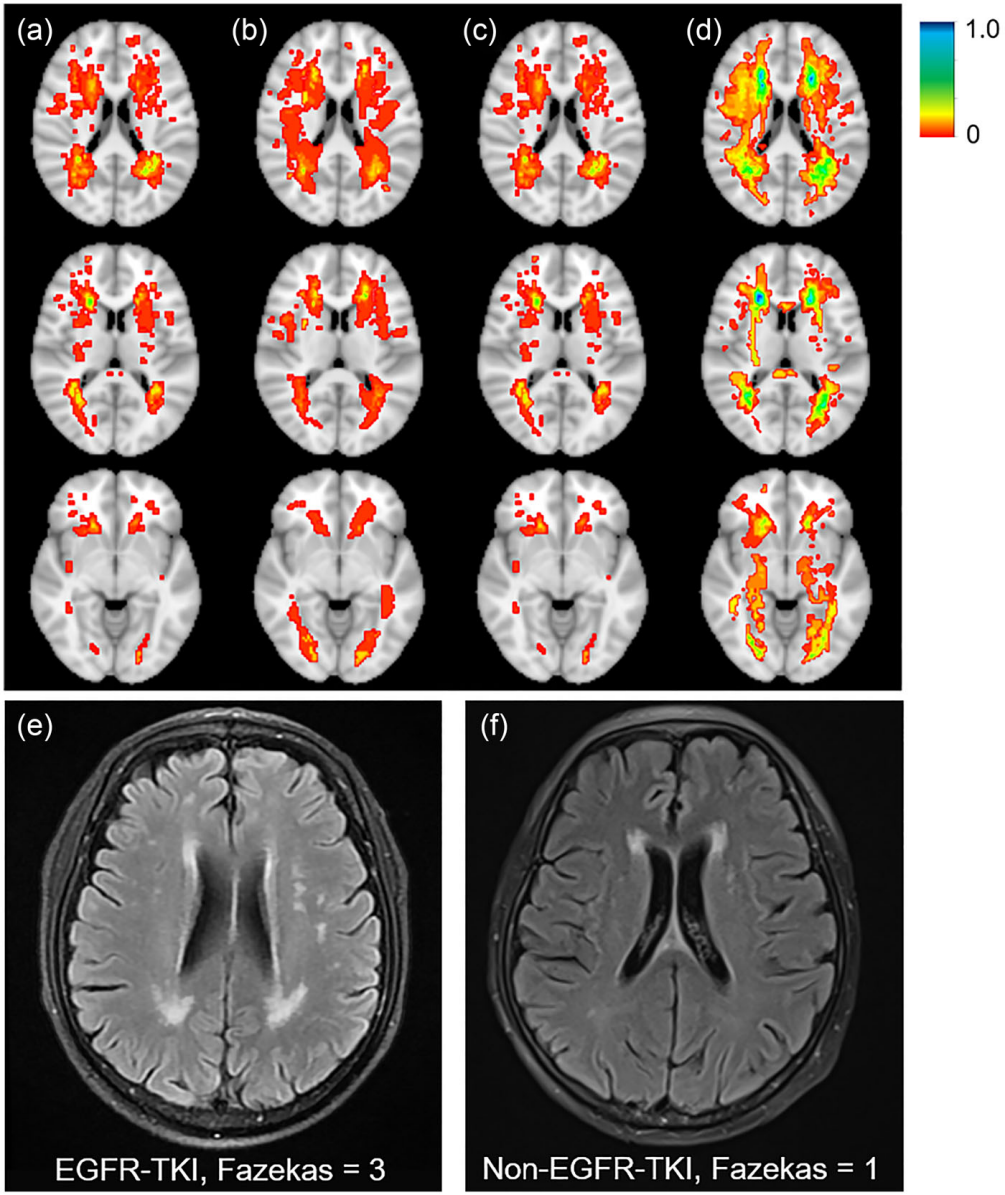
The dynamic changes of white matter hyperintensities white matter hyperintensities (WMH). (a) Spatial distribution of baseline WMH in patients who had not received epidermal growth factor receptor tyrosine kinase inhibitor (EGFR‐TKI) treatment. (b) WMH in the patient had not received EGFR‐TKI at the 2‐year follow‐up. (c) Baseline WMH in patients had received EGFR‐TKI. (d) WMH in the patient had received EGFR‐TKI at the 2‐year follow‐up. T2 Flair images from magnetic resonance imaging (MRI) scans of (e) a 64‐year‐old man with non‐small cell lung cancer (NSCLC) taking EGFR‐TKI 1 year before the scan, a total Fazekas score of 3 (2 for periventricular and 1 for deep) and (f) a 61‐year‐old man has not received EGFR‐TKI with NSCLC, no known vascular risk factors, with a total Fazekas score of 1 (1 for periventricular and 0 for deep).
